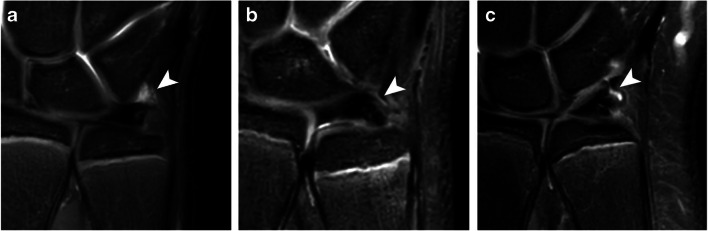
Coronal proton-density weighted fat-saturated MRI showing the a conical shaped prestyloid recess (arrowhead), b tubular shaped prestyloid recess (arrowhead), and c saccular shaped prestyloid recess (arrowhead)
